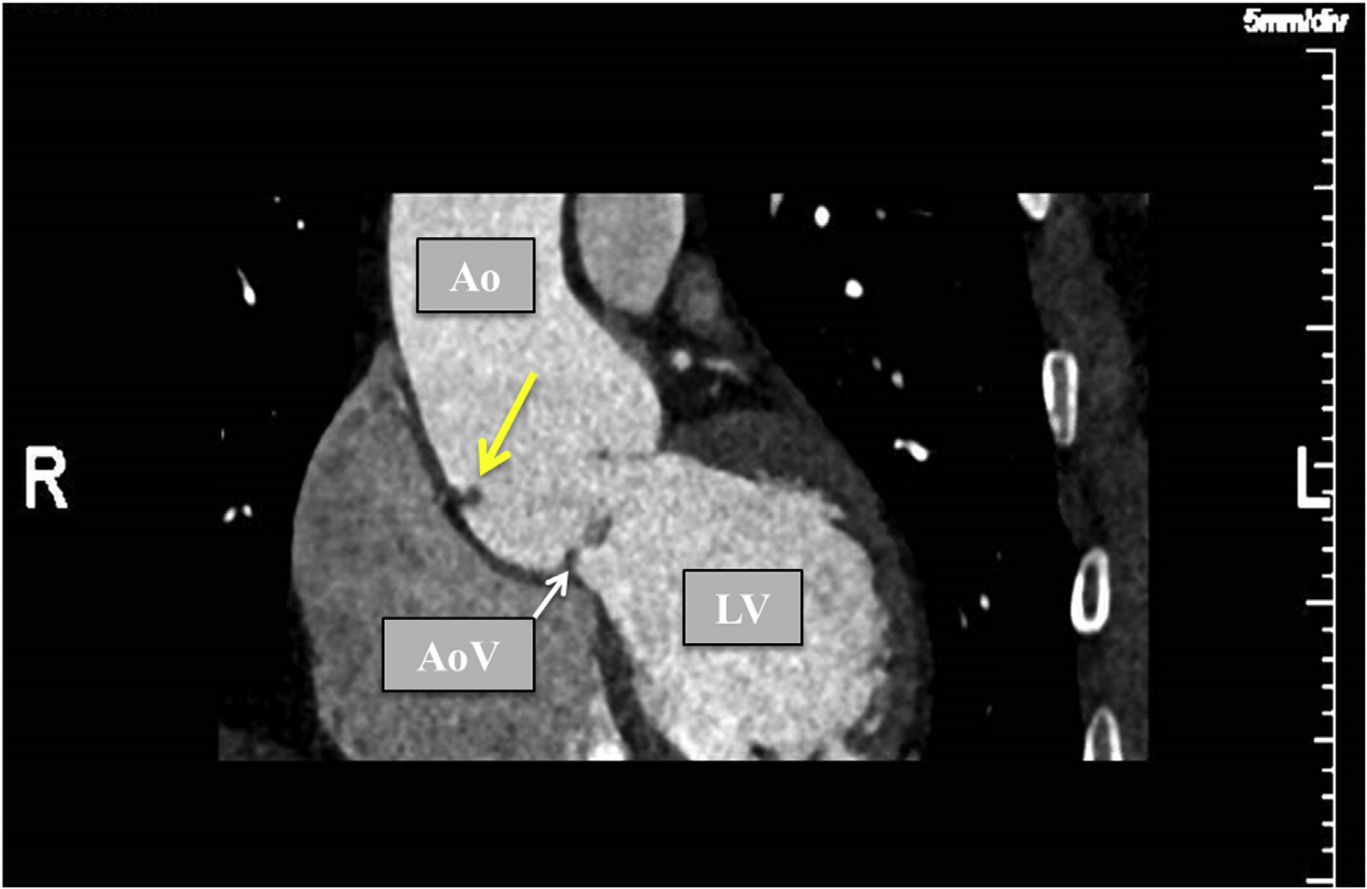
CT scan with arterial contrast enhancement showing the remnant of a fibrous strand at the free wall of the ascending aorta (*yellow arrow*). *Ao*, Aorta; *AoV*, aortic valve; *LV*, left ventricle.
